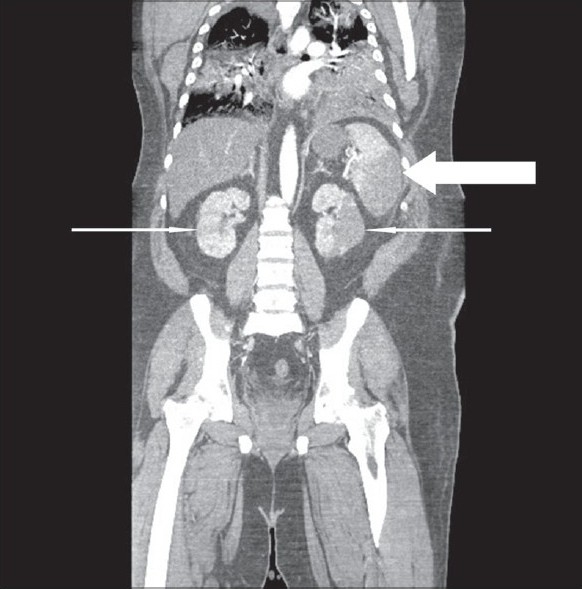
Coronal CTA image showing areas of low attenuation representing infarction within the spleen (thick arrow) and bilateral kidneys (thin arrows).
